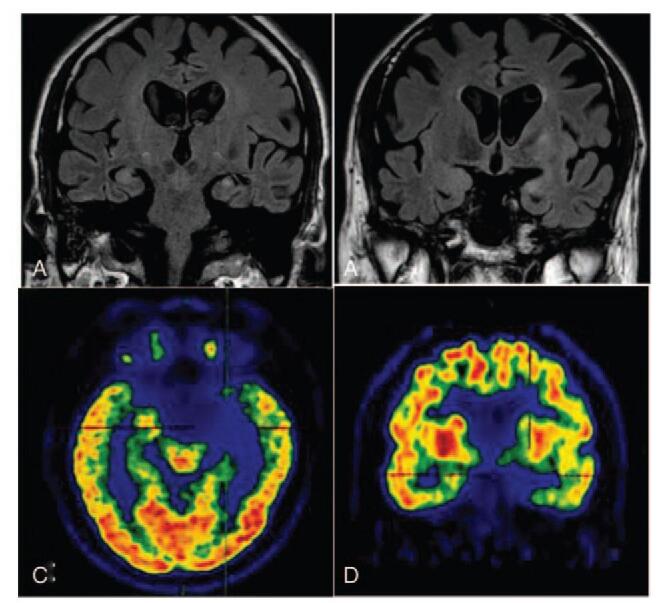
Patient's neuroimaging. (A) T2W FLAIR showed the abnormal hyperintensities in the left medial temporal and hippocampus. (B) T2W FLAIR showed moderate atrophy of the hippocampus with MTA level 3. (C) PET/CT showed the partial absence of radioactivity in the left medial temporal cortex and hippocampus. (D) PET/CT showed the radioactivity is obviously less than the opposite side in the left caudate nucleus head. CT = computed tomography, MTA = medial temporal lobe atrophy, PET = positron emission tomography, T2W FLAIR = T2-weighted fluid-attenuated inversion recovery.
